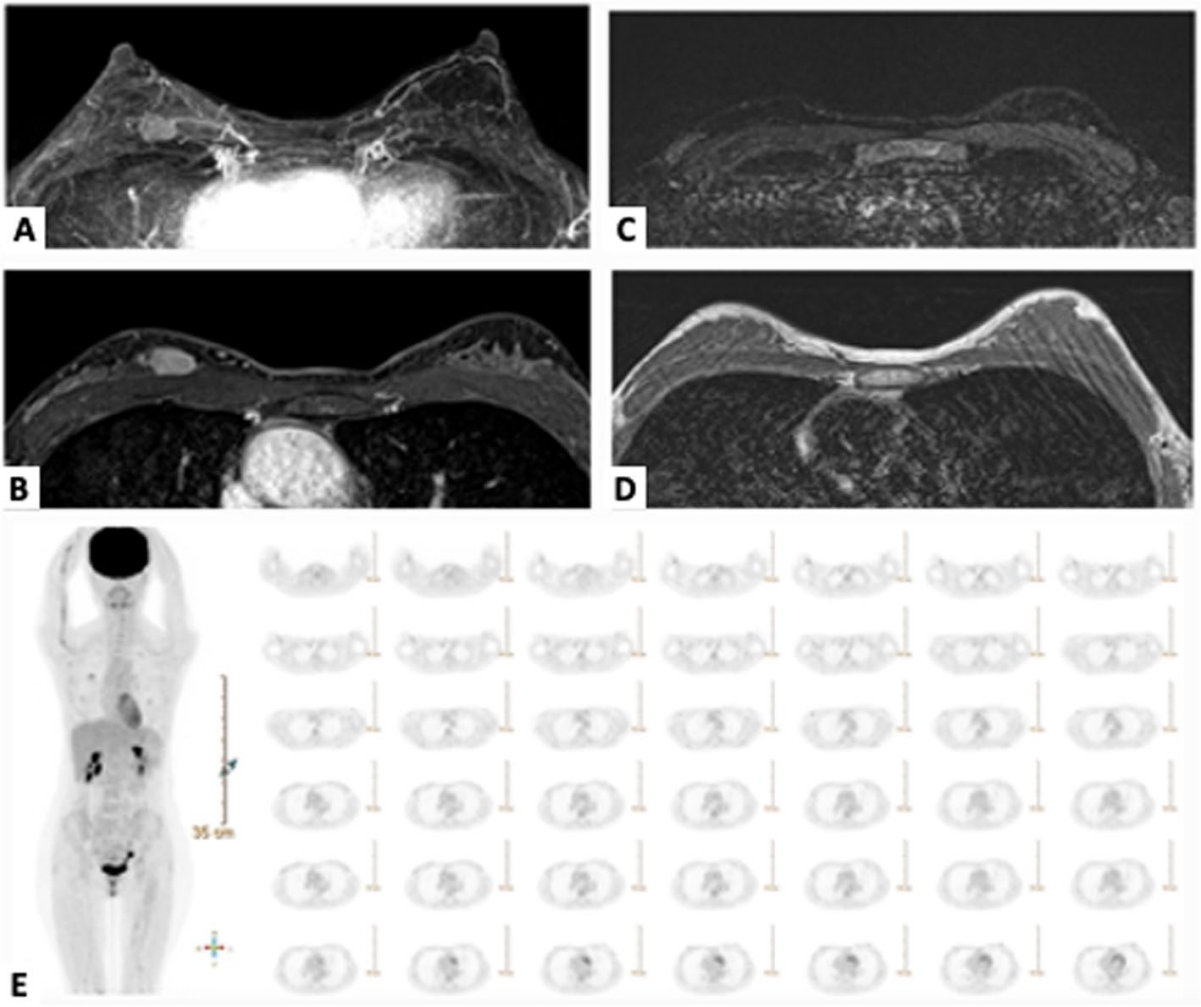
Patient with a discordance between imaging results and pathological results. A 48-year-old female patient with invasive ductal carcinoma in the right breast. A–B Breast MRI showed a 2-cm round mass. C–D Axial T2WI and T1WI images showed no enlarged lymph nodes. E PET/CT demonstrated intense FDG hypermetabolism in the right breast (*SUV*: 2.29/2.81 on early/delayed images), suggesting breast malignancy and a nodular FDG hypermetabolism in the right axilla (*SUV*: 1.68/1.82), suggesting axillary lymphadenopathy. The final pathological report revealed invasive ductal carcinoma with three metastatic lymph nodes (3/19)
